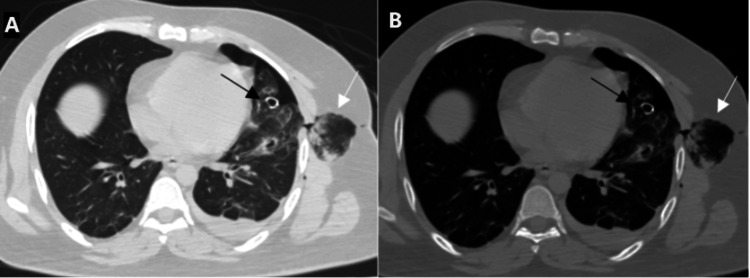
Axial chest CT image with lung window (A) and bone window (B) setting shows the loculated area of air density with lung markings within it in the subcutaneous plane of the left hemithorax (white arrow). This appears to be in continuity with the lung. Associated hemopneumothorax is also seen. The ICD is seen "end on" (black arrow). ICD: implantable cardioverter defibrillator
